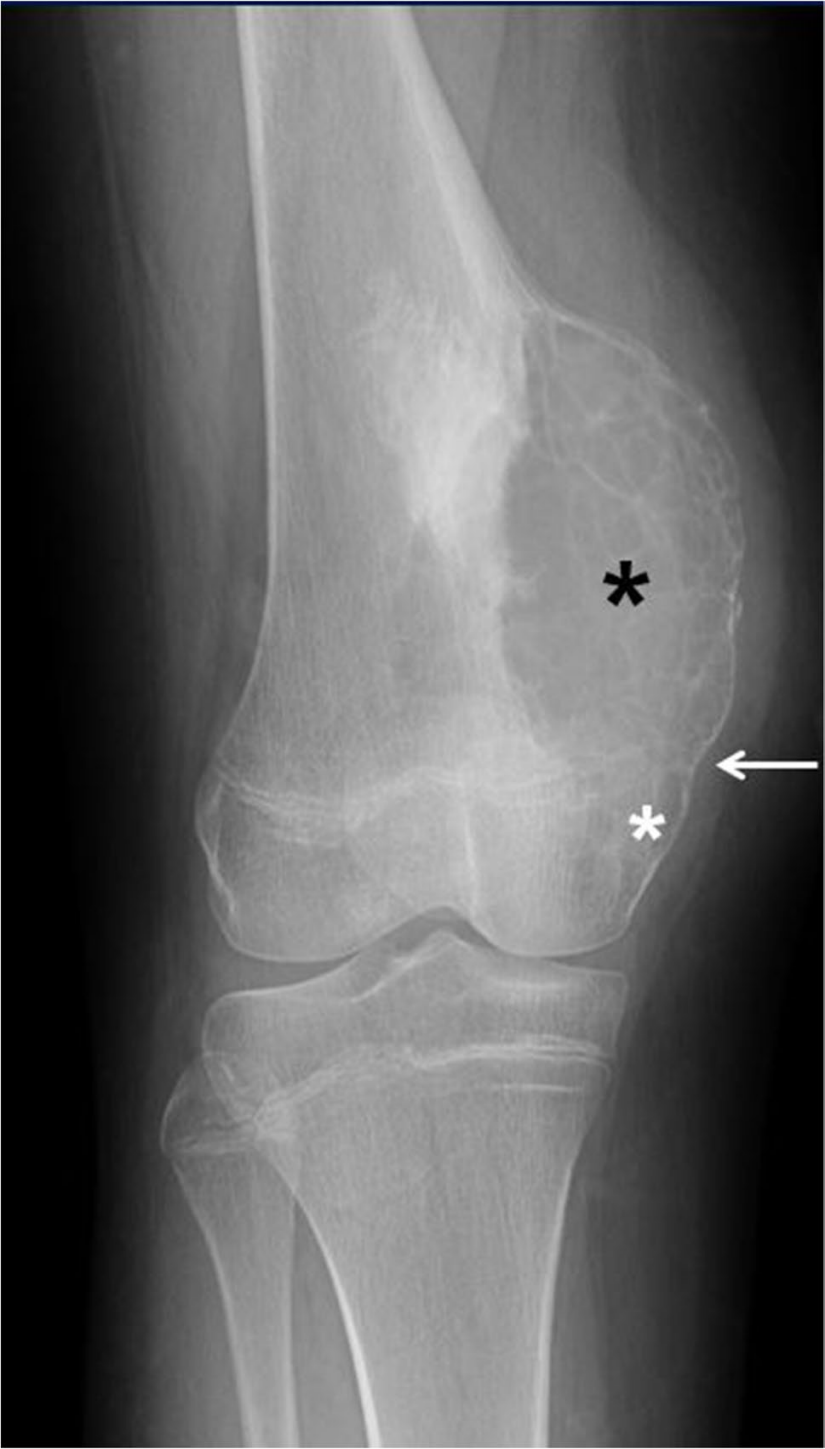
Epiphyseal extension of an aneurysmal bone cyst of the left distal femur in a 14-year-old girl. An anteroposterior radiograph of the left femur shows a well-defined, lytic, expansile metadiaphyseal lesion (*black asterisk*) with multiple internal septations causing cortical thinning. There is clear epiphyseal extension (*white asterisk*) of the lesion across the physis (*arrow*)
